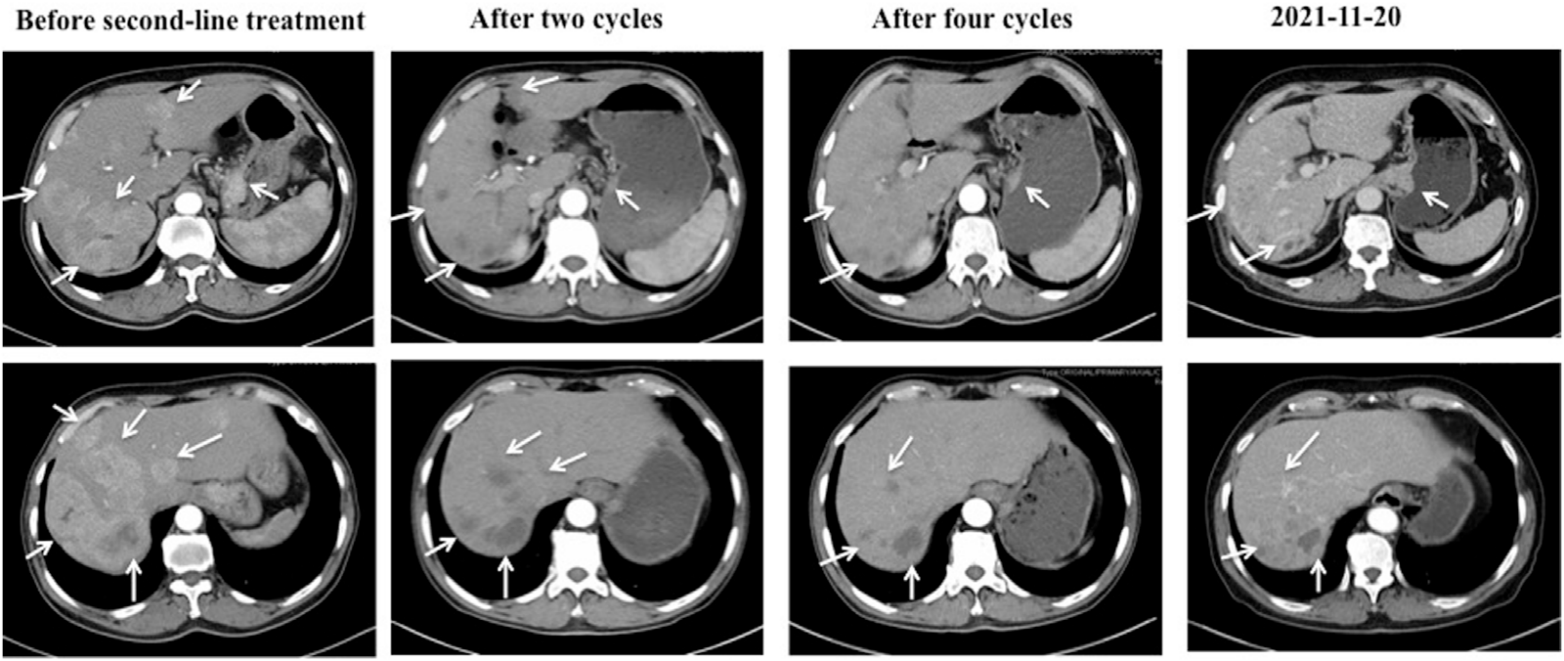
The CT images of the cardiac cancer and hepatic metastases during second-line treatment with apatinib and paclitaxel. Before second-line treatment, the CT showed markedly enlarged cardiac tumor and multiple hepatic metastases with abundant blood supply. The average CT ratio (the density of the liver metastases/the density of abdominal aorta) during arterial phase on CT imaging was 79/210. After two cycles of second-line therapy, the cardiac tumor and hepatic metastases showed a response of partial response (PR), and the ratio was 38/281 with an obviously decreasing of the degree of enhancement. Four cycles later, the efficacy remained PR. The recent CT examination was on 20 November 2021, which showed the disease remained an efficacy of PR.
